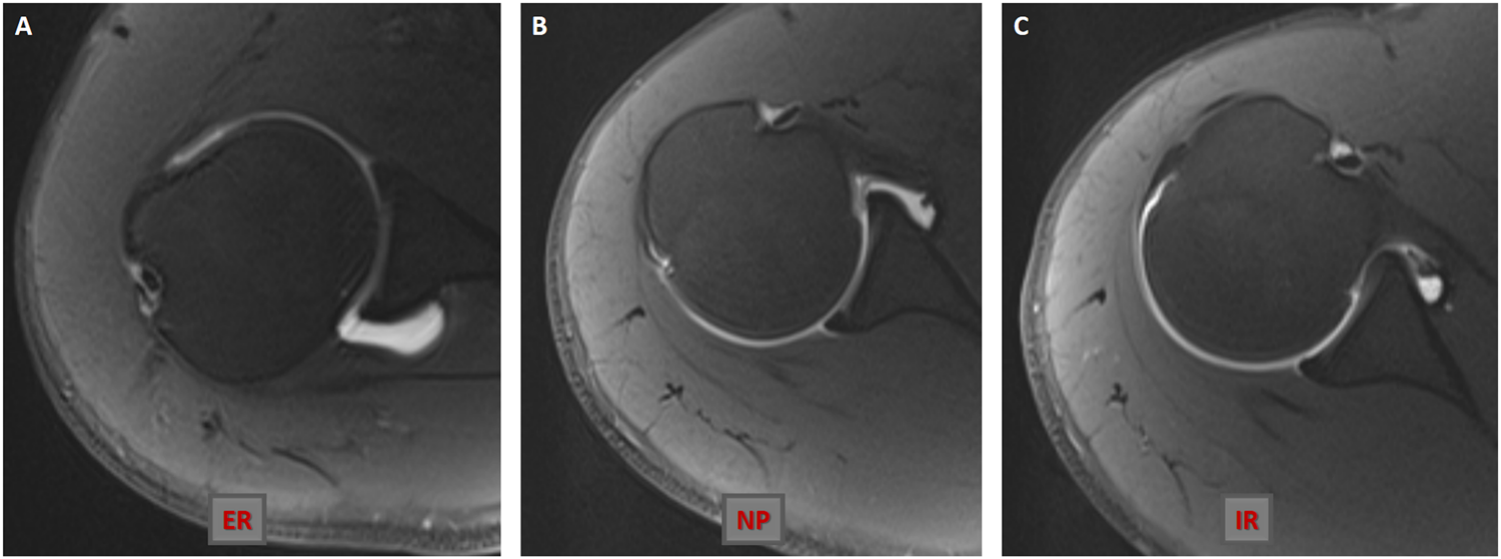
Axial fat-suppressed turbo spin echo (TSE) T1-weighted MR arthrograms (A–C) obtained at the same level show a normal arthrographic anatomy of the posterior shoulder joint capsule in the external rotation, neutral position, and internal rotation of the ipsilateral arm. ER, external rotation; IR, internal rotation; NP, neutral position
